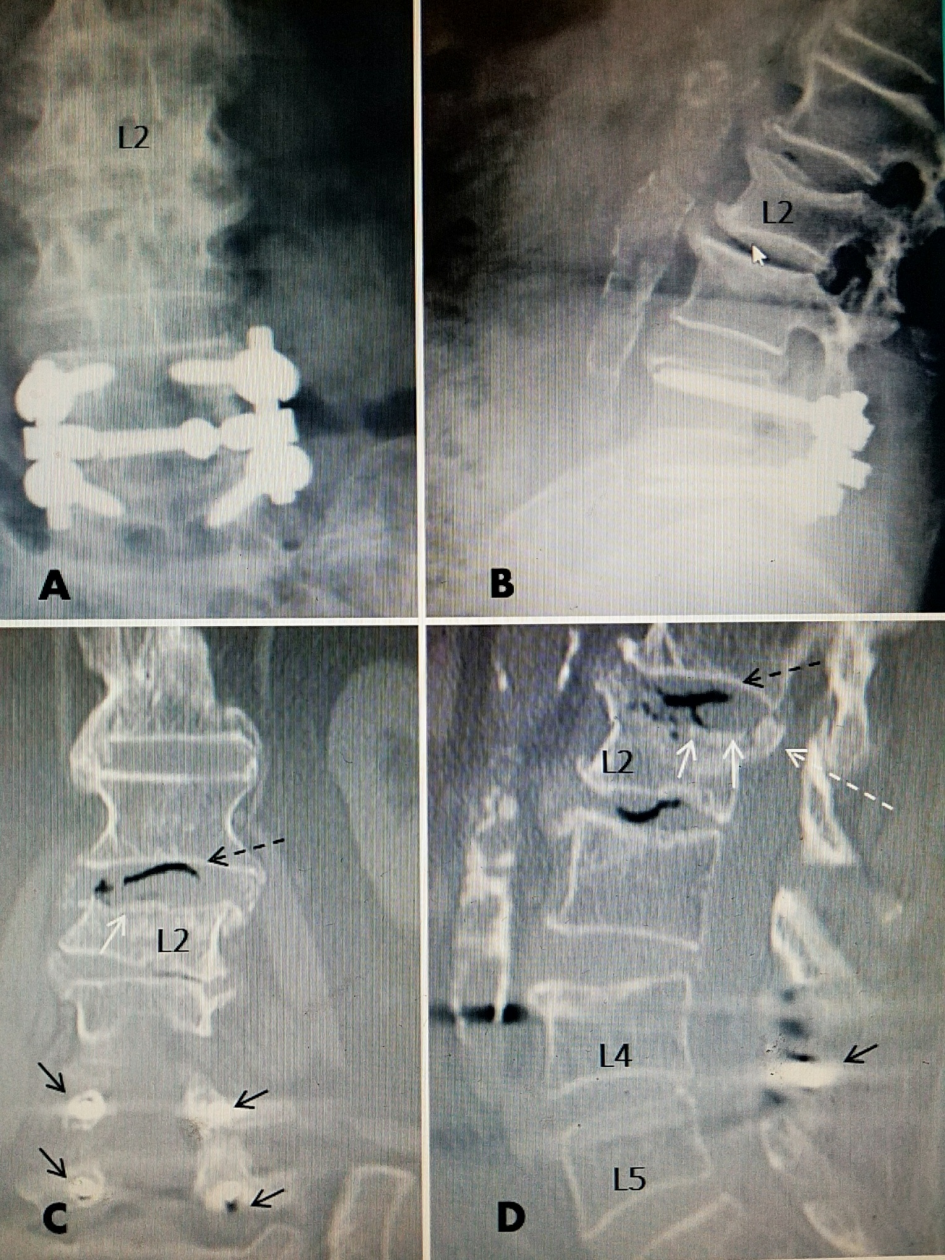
L2 compression fracture after fall above previous L4-5 pedicle fixation A, B: Anterior-posterior (AP) and lateral films showing rigid L4-5 fixation and superior endplate fracture L2. C: Coronal (C) reconstructed computerized tomography (CT) scan showing in greater detail the collapsed fracture of the superior endplate. There is a black signal in disc space at L1-2 (dotted black arrow). The L4 and L5 pedicle screws can be seen in proper position (solid small black arrows) D: Sagittal reconstructed CT. There is fracture and posterior displacement of the superior endplate into the spinal canal (dotted white arrow). Comminuted fracture of superior endplate of L2 (dotted white arrows). There is necrotic aseptic vacuum change at L1-L2 disc and also at L2-3 disc shown by black signal on CT scan (dotted black arrow). The cross link connecting the two lateral rods is seen between the spinous process at L4-5 (small black arrow).
